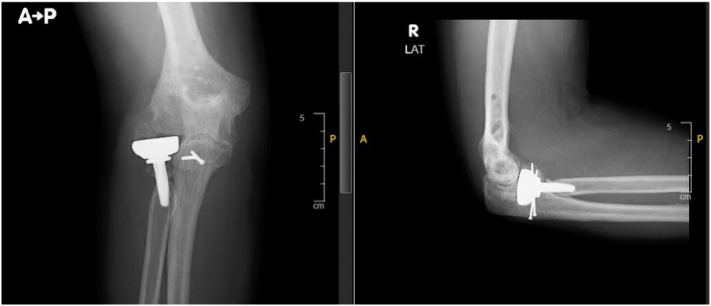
Follow-up X-ray of the right elbow taken after 7 years of the procedures indicating good healing process of the coronoid fracture.
